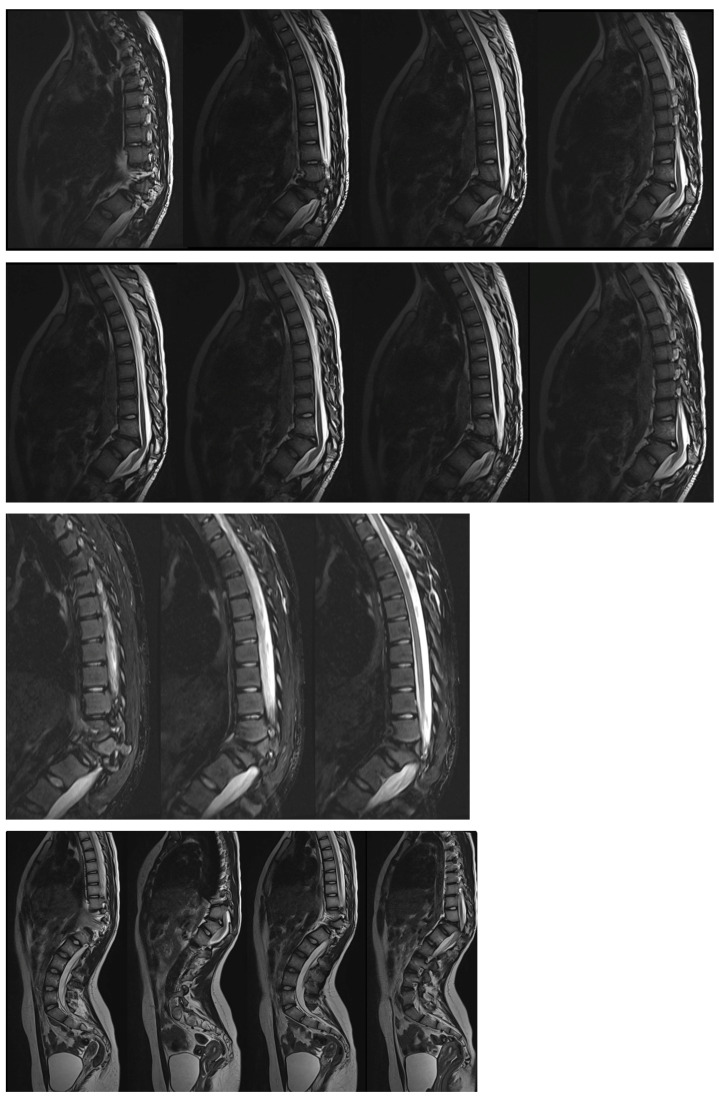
MRI scans showed spinal cord compression in the 16-year-old female before surgical treatment.
